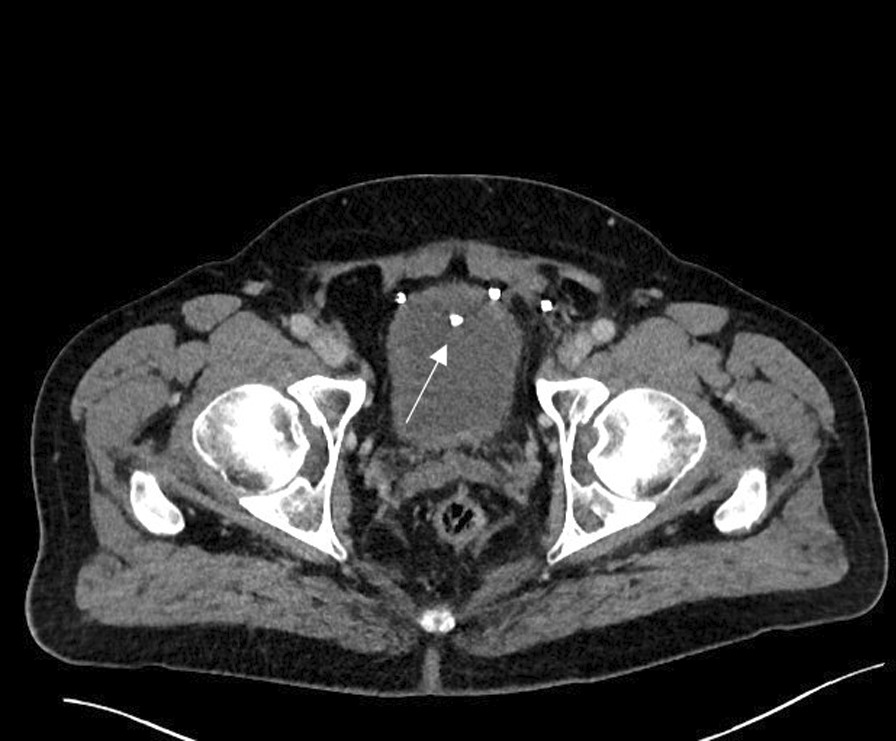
Computed tomography scan showing a stone in the bladder roof indicated by white arrow
